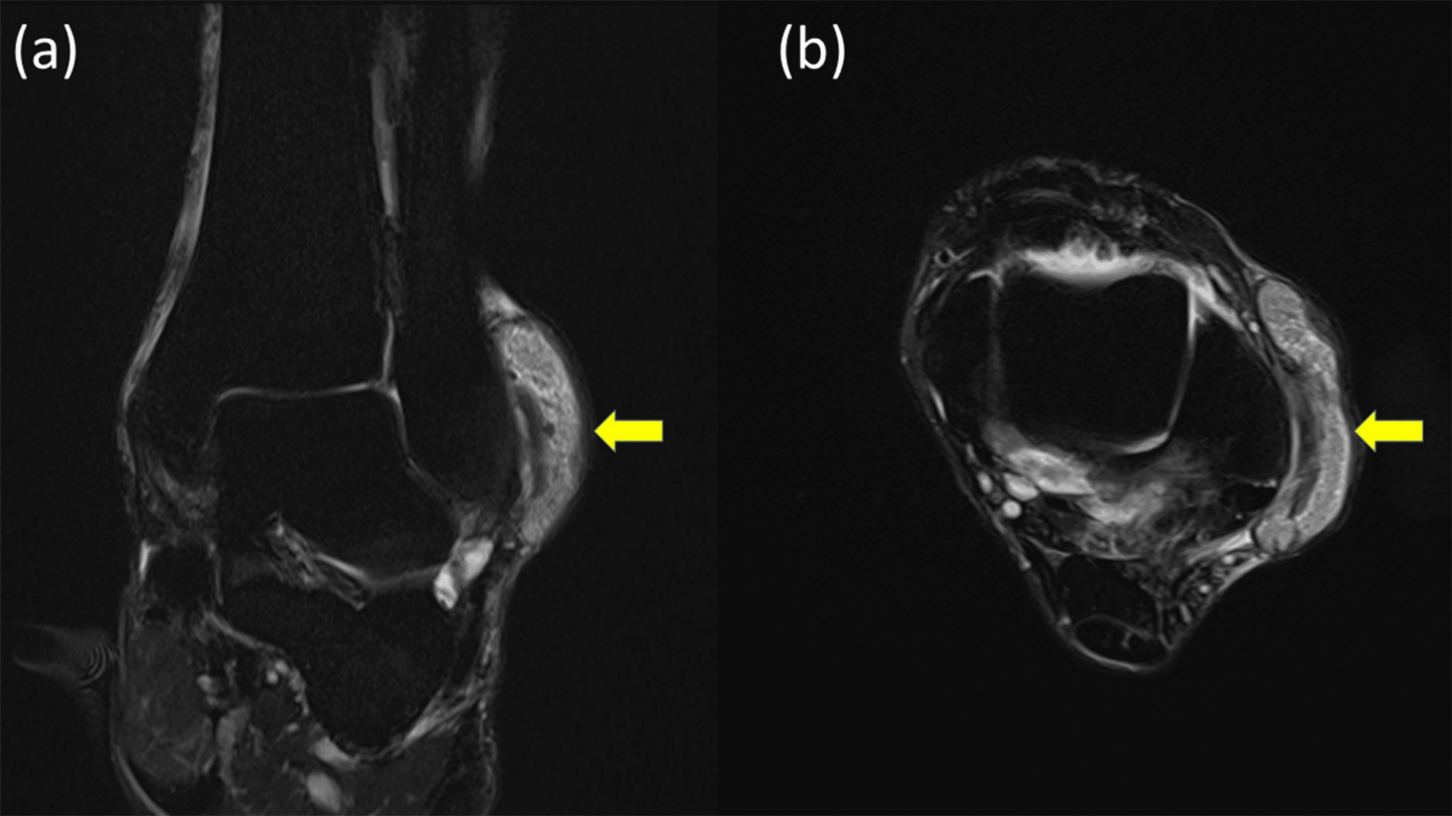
Preoperative magnetic resonance imaging (a) Coronal and (b) axial fat-suppressed T2-weighted magnetic resonance images demonstrate periarticular soft tissue inflammation with joint effusion. Multiple rice body-like structures are observed around the ankle, appearing as hyperintense nodular lesions within the extra-articular soft tissues (arrow). No definitive intra-articular tophaceous mass or articular cartilage destruction is identified.
